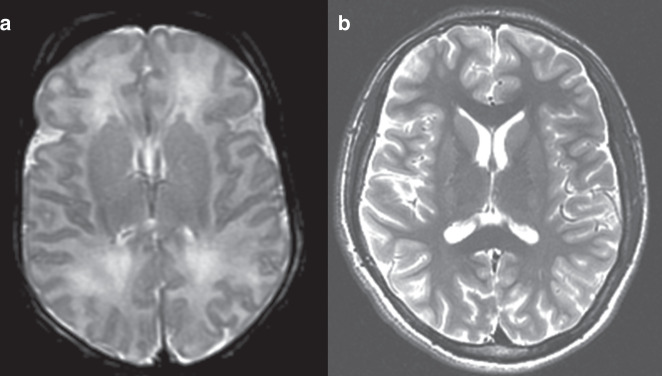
Neonatal and adolescent magnetic resonance imaging (MRI) of patient no. 1. a Axial T2-weighted imaging of the neonatal brain demonstrates T2-hyperintensity in bilateral frontal and parietal white matter in a watershed distribution. There was no associated reduced diffusion (not shown), so injury appears subacute. b Follow-up adolescent MRI through the same level demonstrates normal signal and volume.
